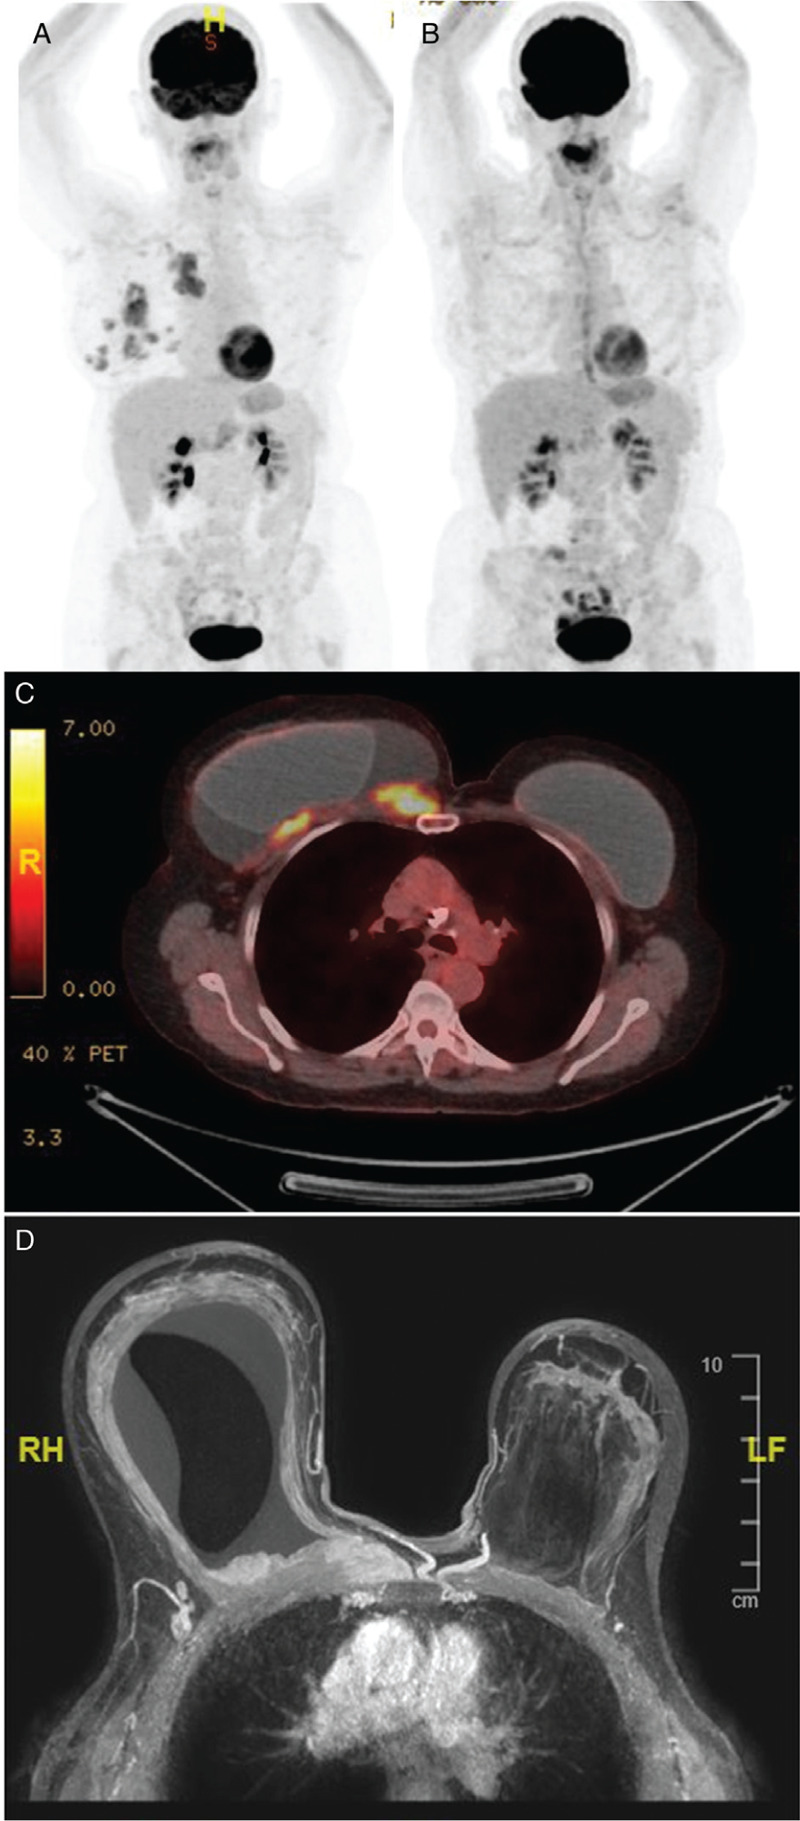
Radiological images. PET MIP images pre-treatment (A) and following 8 cycles of BV (B) demonstrating a complete metabolic response (Deauville 1). Pre-treatment axial image from PET-CT (C) showing the FDG-avid soft tissue mass and dynamic MIP MRI (D) demonstrating infiltration of the underlying muscle.
